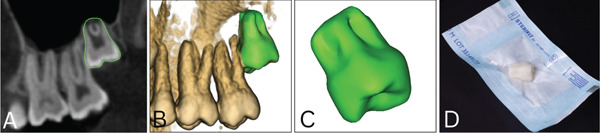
CBCT‐based digital planning and fabrication of a sterilized 3D tooth replica from Case 2. (A) CBCT showing an impacted tooth outlined in green. (B) 3D reconstruction of the jaw with the impacted tooth highlighted in green. (C) Digital 3D model of the tooth. (D) 3D‐printed replica of the tooth on sterile packaging, ready for surgical planning. The replica was sterilized at 121°C in a two‐atmosphere steam sterilization cycle (60 min).
